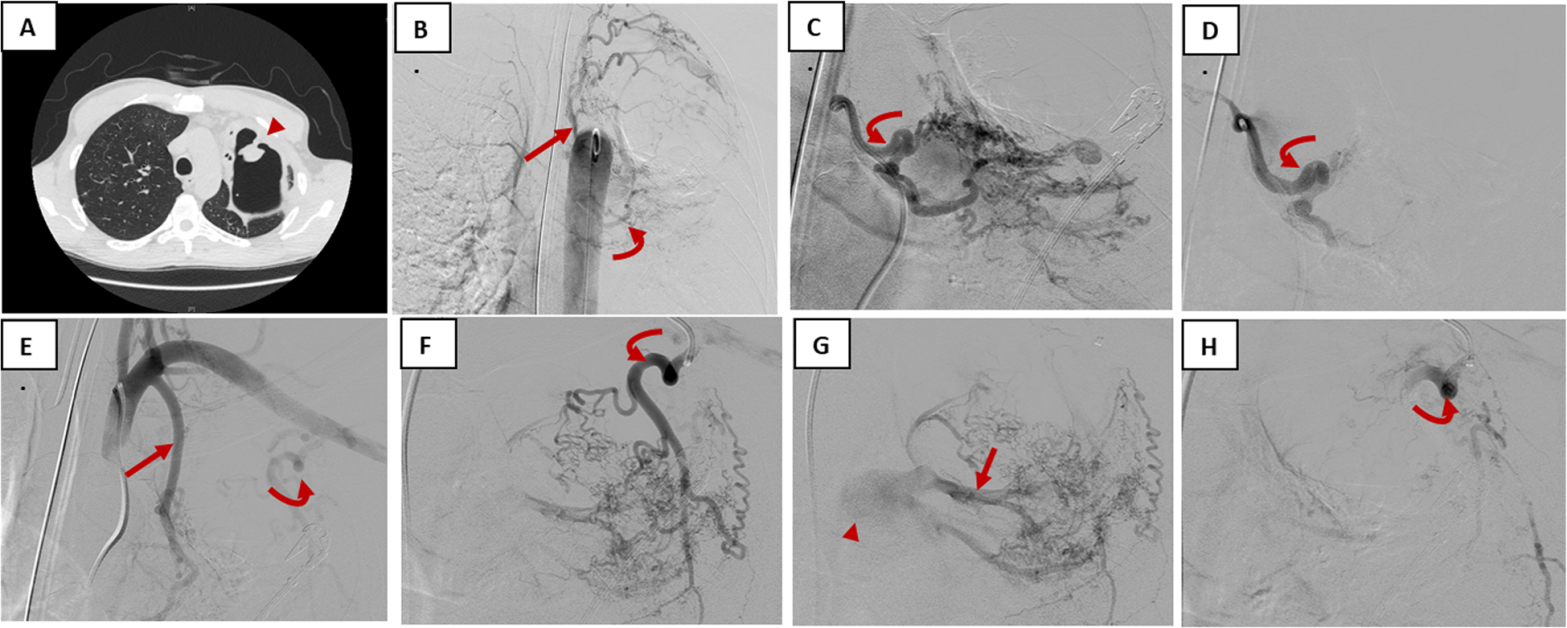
Case presentation. A patient in his late thirties with HIV, tuberculosis (4-drug treatment in 2009), and LUL cavitary lesion with aspergilloma presenting with life-threatening hemoptysis. a Single image from axial computed tomography shows aspergilloma (arrowhead), which was the likely etiology of this patient’s hemoptysis. b Flush thoracic aortogram demonstrates hypertrophied bronchial arteries (curved arrow) and superior intercostal arteries (straight arrow) supplying the aspergilloma. c Representative image of selective angiogram of the larger of the 2 left bronchial arteries shows hypertrophied bronchial artery (curved arrow). d Angiogram of the bronchial artery (curved arrow) post-bronchial artery embolization with 300–500 μm particles (trisacryl gelatin microspheres) via selective microcatheter demonstrates lack of blood flow to the area of the aspergilloma. e Angiogram of the left subclavian artery to evaluate for non-bronchial systemic collaterals demonstrates abnormal neovascularity filling from the left internal mammary artery (straight arrow) and lateral thoracic artery (curved arrow) on the left side. f Representative image of selective angiogram of the lateral thoracic branch on the left. g Angiogram shows inflammatory neovascular blush (arrowhead) with shunting to the main pulmonary artery (straight arrow). h Post-procedural angiogram with 500–700 and 700–900 μm particles demonstrates successful embolization of the lateral thoracic branch on the left.
